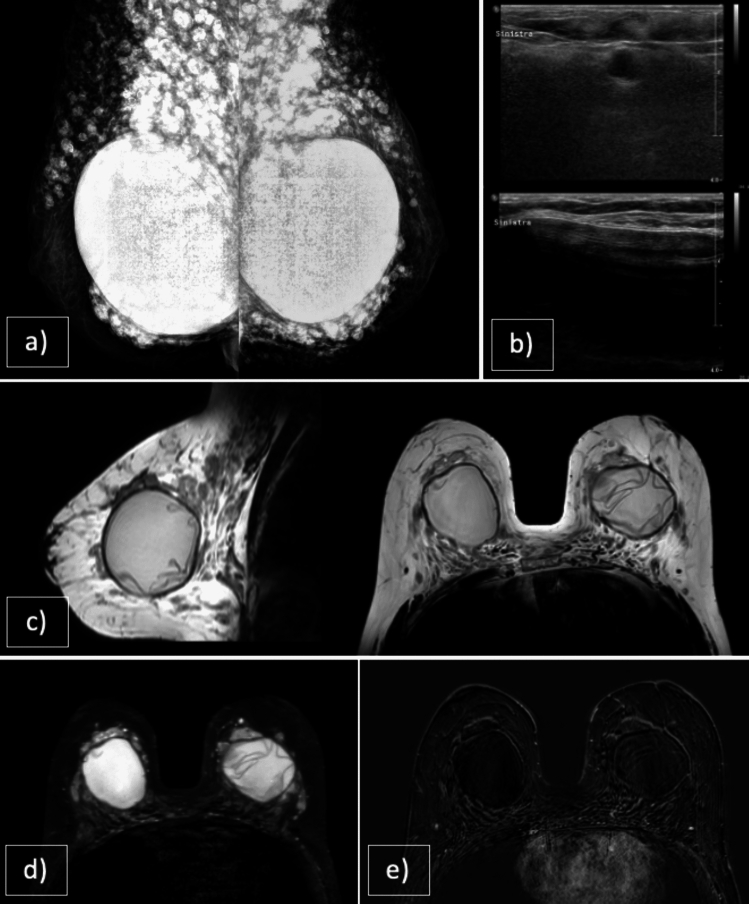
a MX, b US, c sagittal and axial T2WI, d silicon-only sequence and e CE T1WI. Breast augmentation with silicone implants and several injection of silicone material was performed in a 40-year-old MtF patient. MX and US reveal extensive coverage of the prosthetic implants by the silicone injection, which appears radiopaque on MX and produces a diffuse *snowstorm-like* effect on US. To further assess the patient, an MRI was conducted, which clearly demonstrated an intracapsular rupture of the left implant, characterized by the *linguine sign*. No pathological enhancement was observed, excluding an otherwise invisible malignancy. The patient was entrusted to the plastic surgeon
